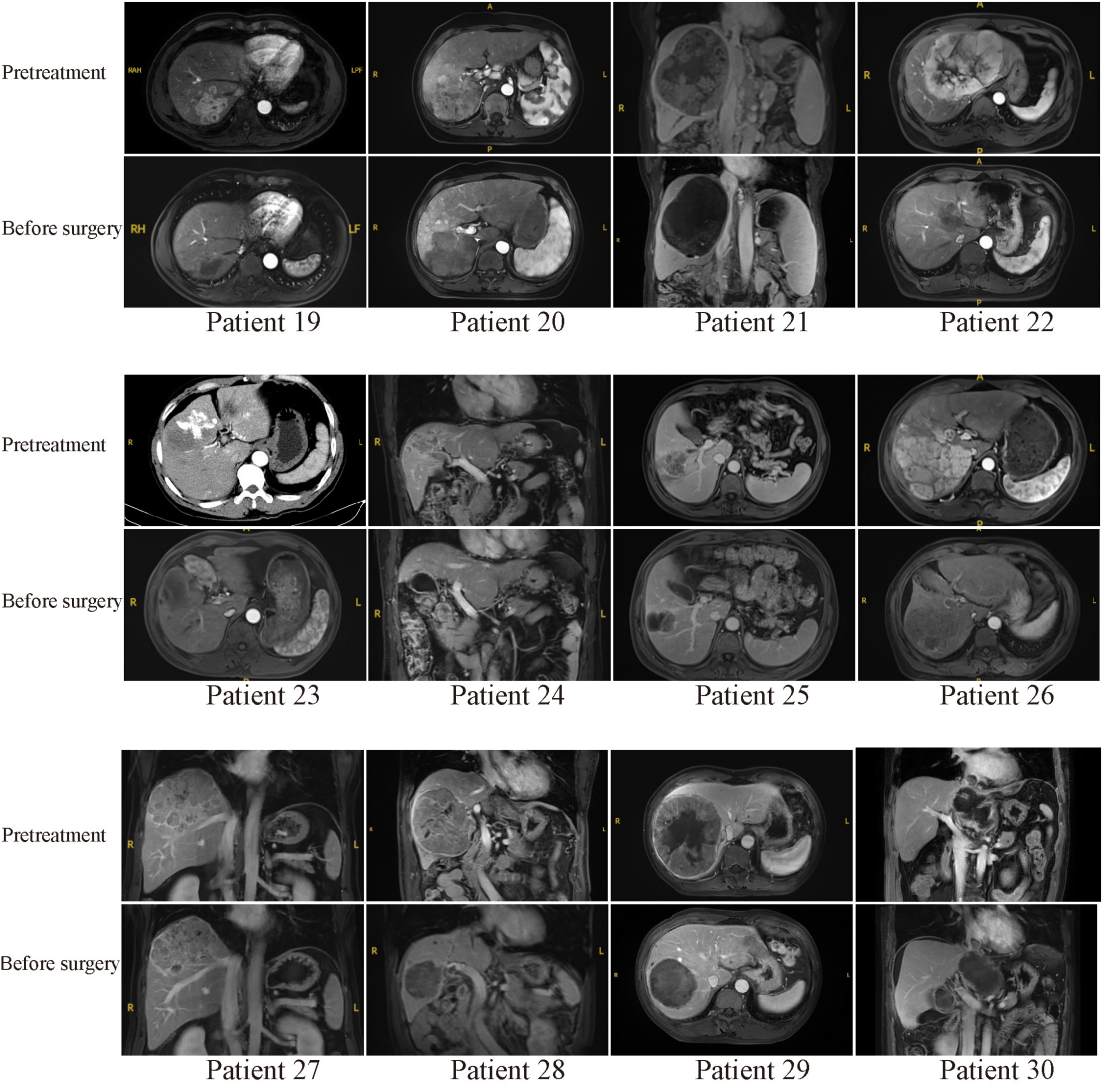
Pretreatment and preoperative MR or CT scans of patients 19-30. Patient 23 received emergency hepatic arterial chemoembolization due to rupture of hepatocellular carcinoma in other hospital before combination therapy.
